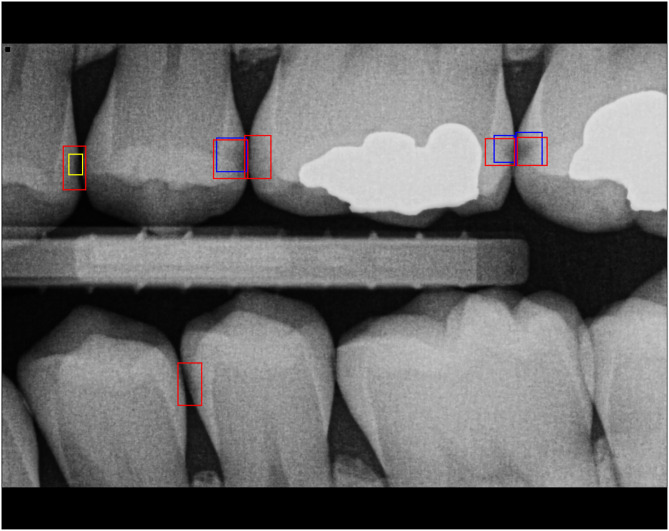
Sample bitewing radiograph with annotations of a student annotator (red), reference standard majority set S (blue), and minority set S′ (yellow).
